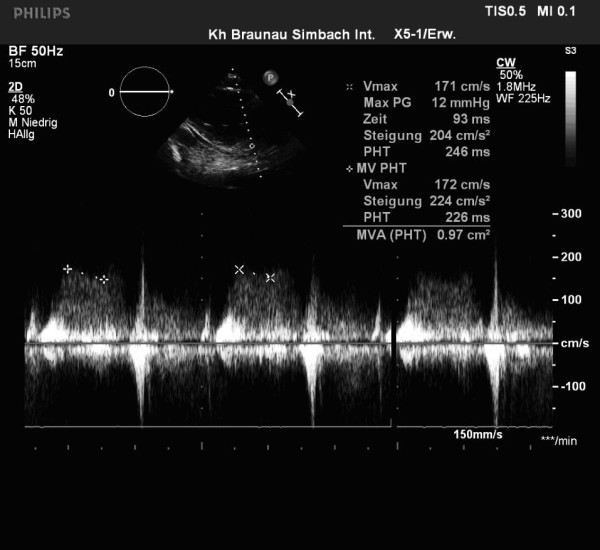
CW - spectral Doppler tracing indicating severe mitral stenosis.
